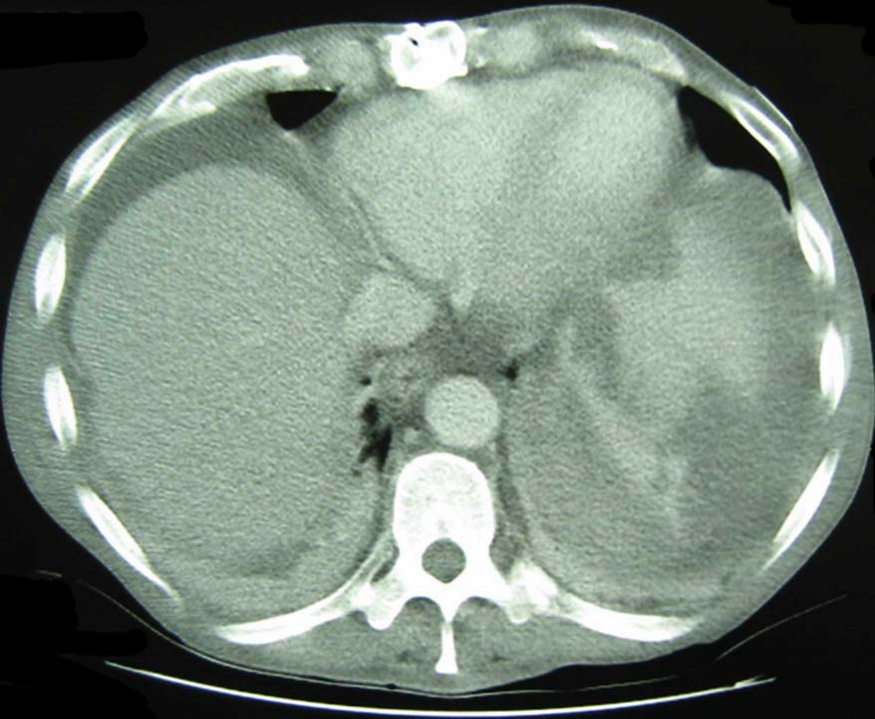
Computed tomography (CT) scan. The CT scan demonstrates hemoperitoneum and a grade IV splenic laceration in our patient, who had no history of trauma.
